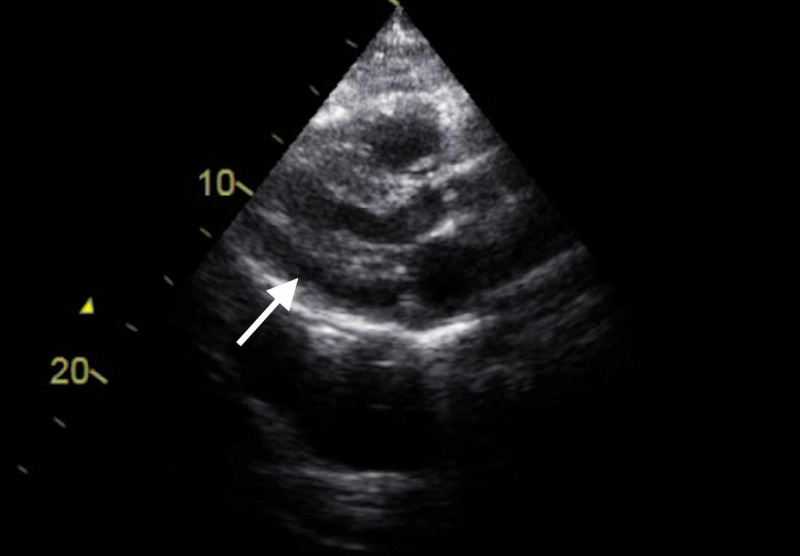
Echocardiography shows pericardial effusion (white arrow).
